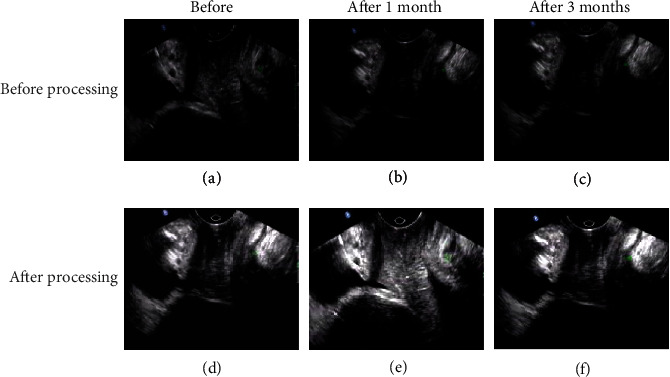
Ultrasound images of the control group at each time period.
